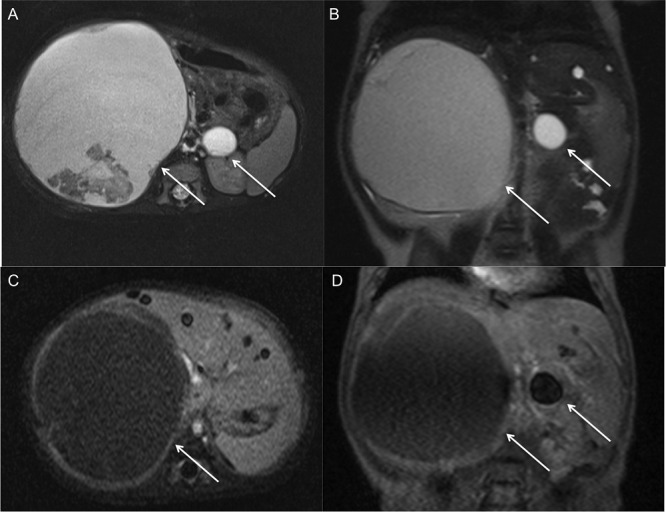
Figure 1: MRI examination of the patient. A, B) Both lesions (arrows) are hyper-intense on axial and coronal T2-weighted sequence. Note the calcification in the postero-inferior part of the right-sided adrenal mass. Liver lesions have the same characteristic as adrenal masses. C, D) Adrenal lesions (Arrows) have thickened walls with contrast enhancement. Note that multiple cystic lesions in the liver do not show contrast enhancement.
